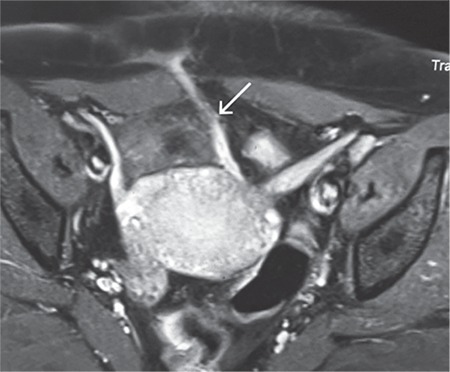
Post-contrast magnetic resonance image showing the enhancing fistulous tract (arrow) from the right end of the abdominal incision traversing through the abdominal cavity to the uterine wall
